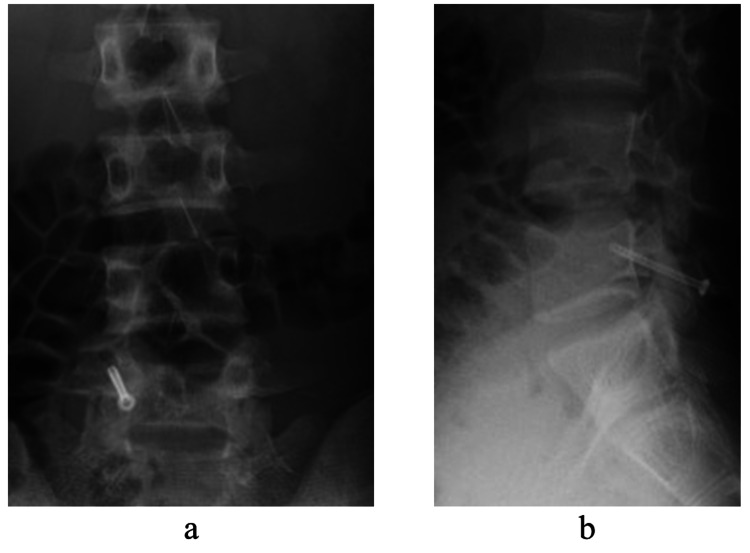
Postoperative plain radiographs Plain radiographs just after surgery (a, b).
